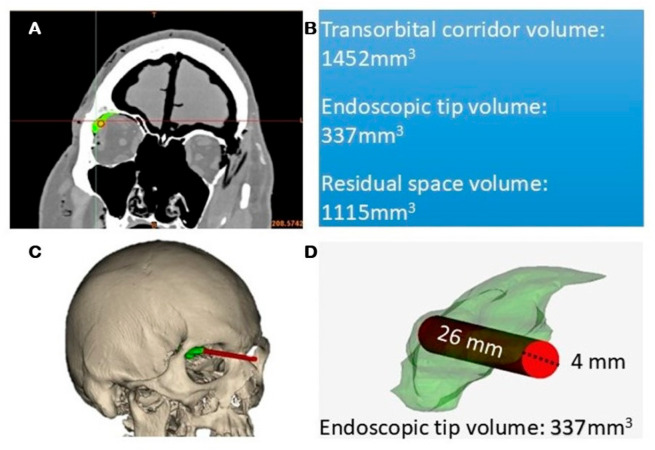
CT reconstruction of the transorbital corridor volume demonstrates the hindrance of the endoscopic tip during the orbital phase. (A): CT scan images, (B): quantitative analysis; (C): 3D scan reconstructions; (D): volumetric measurements.
